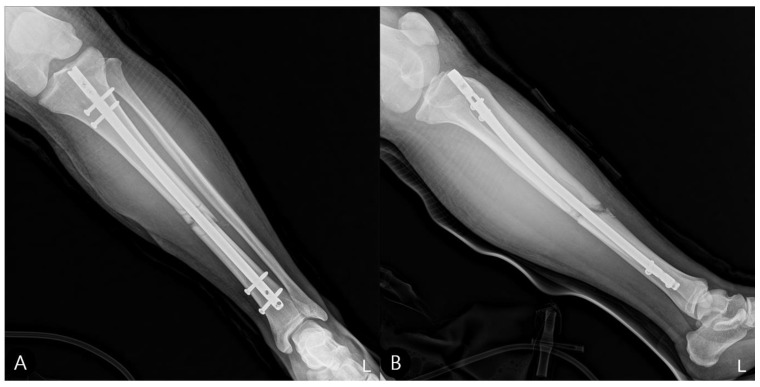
Postoperative tibia radiographs following intramedullary nailing. (A) AP and (B) lateral views showing satisfactory fracture alignment and stable fixation. “L” indicates the left side.
